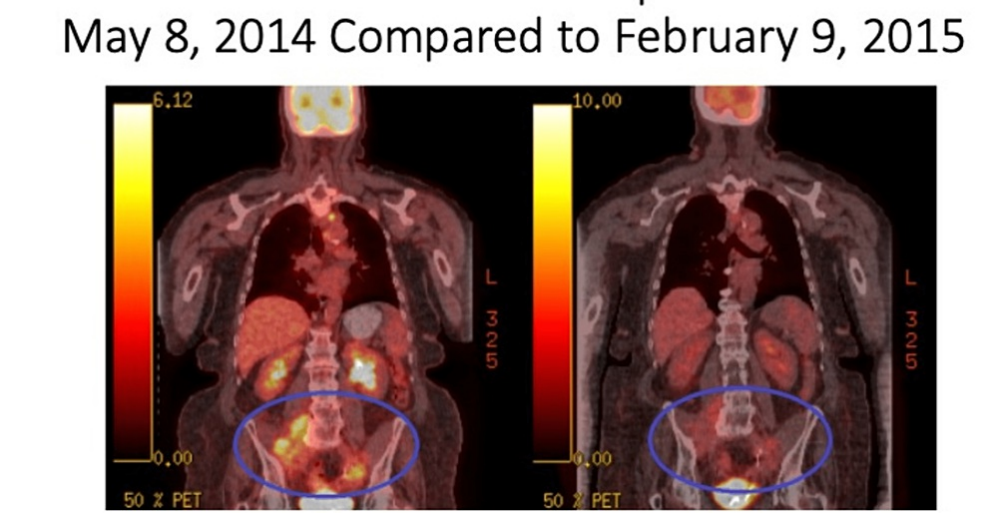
PET-CT Comparisons Before and Six Months After Completion of HEATT® and Chemotherapy Regimens Comparison between PET-CT prior to HEATT® (left side) and after both HEATT® and chemotherapy (right side).
